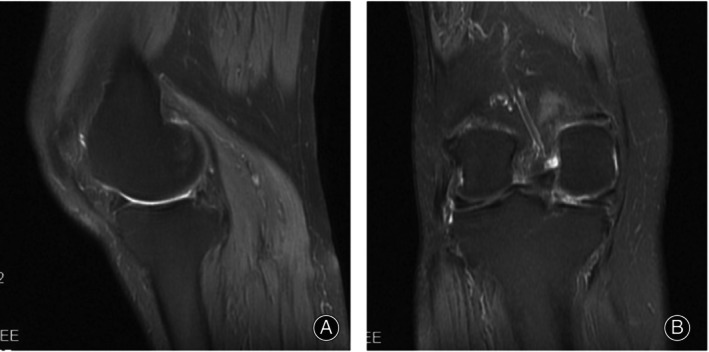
Magnetic resonance imaging (MRI) of the affected knee joint at 1 year follow‐up. (A) Sagittal MRI showed the presence of solid tissue at the posterior root attachment. (B) Coronal MRI showed that the medial meniscus was located between the medial tibiofemoral joint, with no obvious protrusion deformity.
